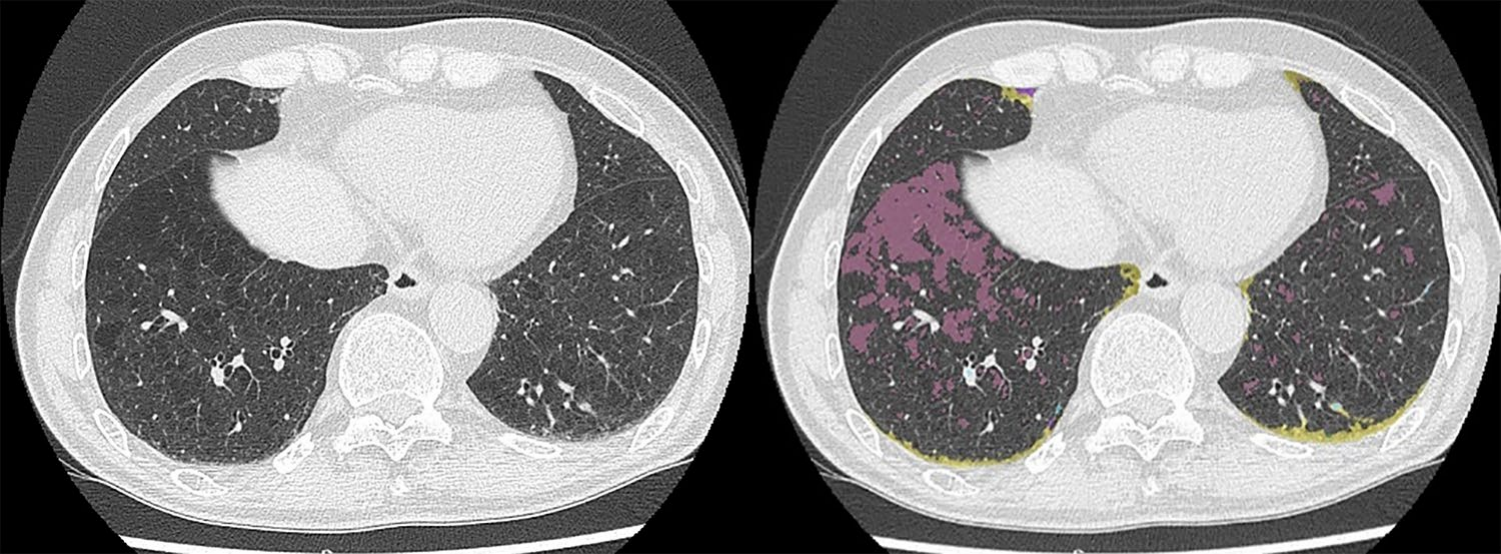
73-year-old male patient with suspected COVID-19 pneumonia and diagnosed as negative on RT-PCR. (L to R: thin-section CT to CAST result) On thin-section CT, no ground-glass opacity or consolidation was observed in either lung. Low attenuation areas assessed as emphysema were observed in both lungs, and reticulations within the peripheral zone in both lungs. CAST shows emphysema as purple and reticulation as yellow. The PCR test also showed this case as “negative”. All chest radiologists and the CAST software assessed this case as “negative for pneumonia” and “negative case”, so that this case was judged to be true-negative
